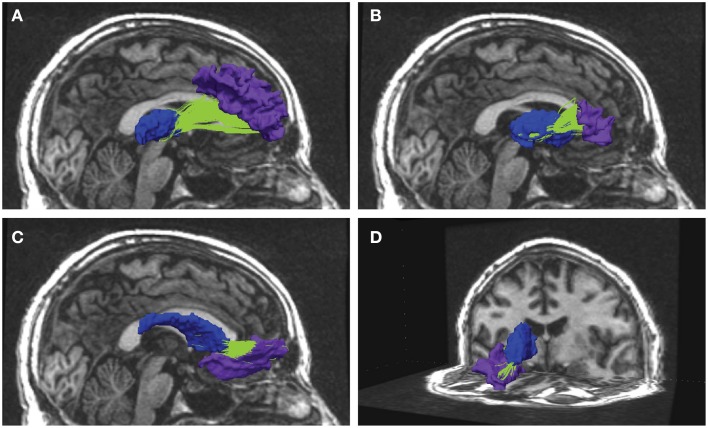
Examples from reconstructed frontal-subcortical fiber bundles in right hemisphere. The images show four different frontal-subcortical fiber bundles reconstructed in one of the participants from the multimodal MRI recordings at Wave-2. The selected WM tracts (in green) are co-registered with and superimposed on the 3D T1-weighted images, where the source and target regions obtained from FreeSurfer segmentation are color-coded. Blue segment represents subcortical seed region and purple segment represents frontal WM target region. Fiber bundle connections in the right hemisphere are running between: (A) thalamus and rostral middle frontal region; (B) putamen and the pars triangularis; (C) caudate nucleus and lateral orbitofrontal region; (D) caudate nucleus and medial orbitofrontal region.
